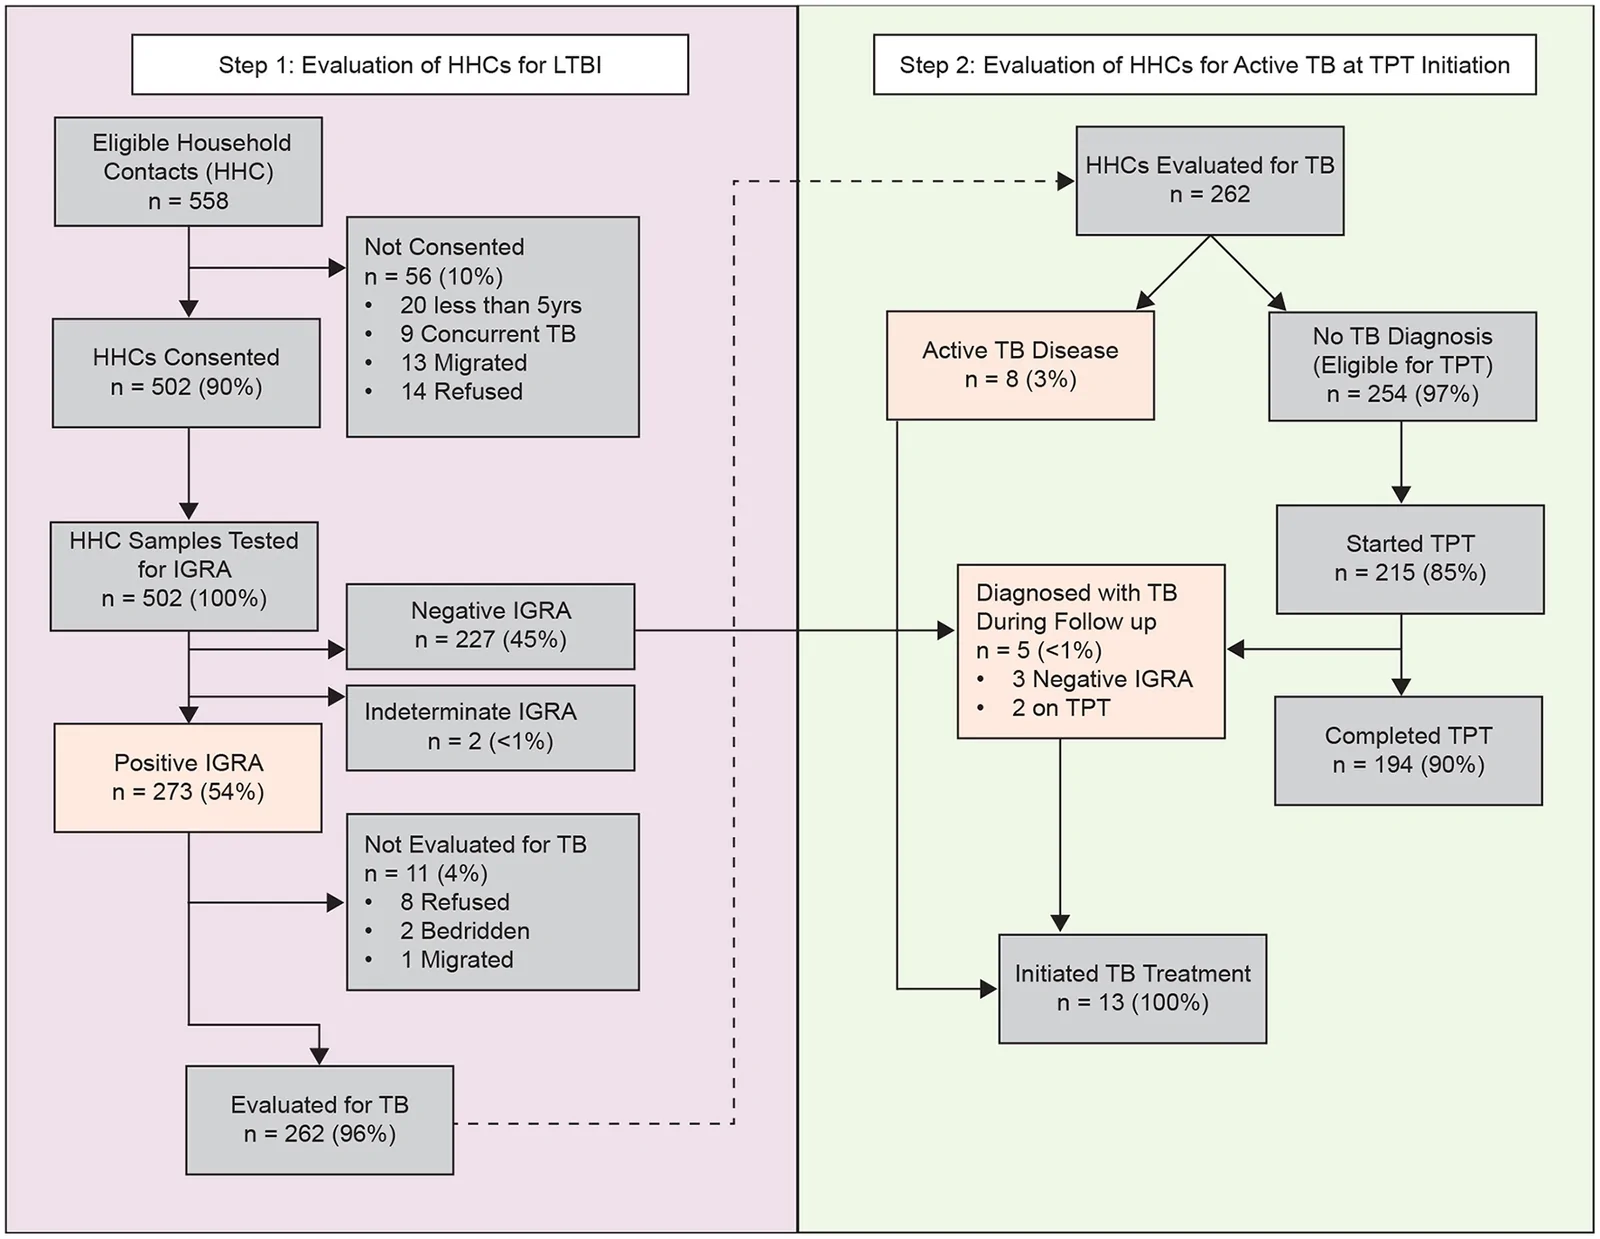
Identification, enrolment, evaluation, follow-up outcomes of household contacts of pulmonary tuberculosis, Mumbai, India—September 2021–31 December, 2023. Participants evaluated for active TB disease included those with positive IGRA results and received a chest radiograph. Highlighted boxes (orange) indicate primary outcomes (TB infection or active TB disease). HHC, household contact; IGRA, interferon-gamma release assay; TPT, tuberculosis preventive treatment.
